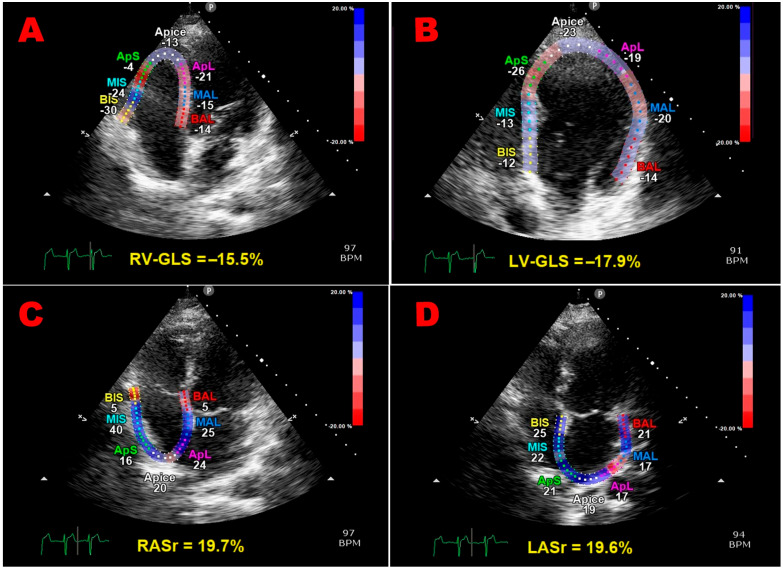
Representative examples of right ventricular (A), left ventricular (B), right atrial (C) and left atrial (D) longitudinal strain parameters measured from the apical four-chamber view in a pGDM woman included in the present study. All myocardial strain parameters were reduced in comparison to the accepted reference ranges. GLS, global longitudinal strain; LASr, left-atrial reservoir strain; LV, left-ventricular; pGDM, previous gestational diabetes mellitus; RASr, right-atrial reservoir strain; RV, right-ventricular.
